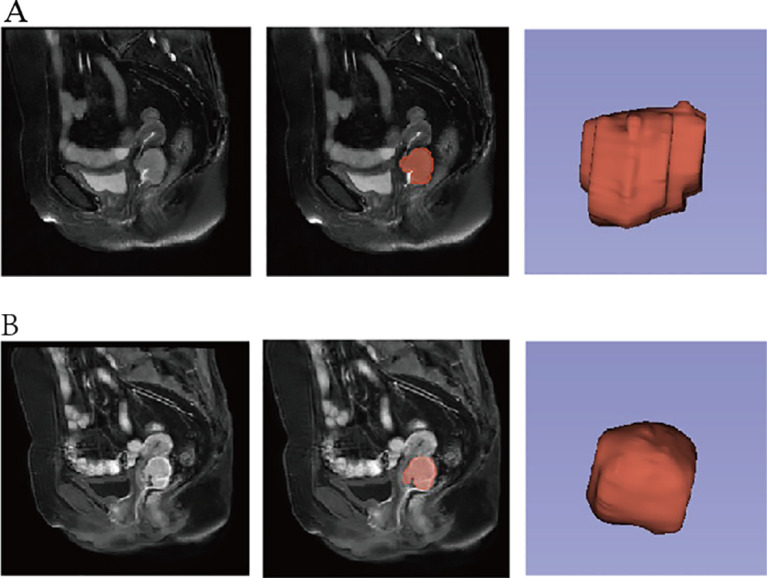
Manual outlining of ROI: (A) sagittal T2WI image and (B) sagittal CE-T1WI image. ROI, region of interest; T2WI, T2-weighted imaging; CE-T1WI, contrast-enhanced T1-weighted imaging.
